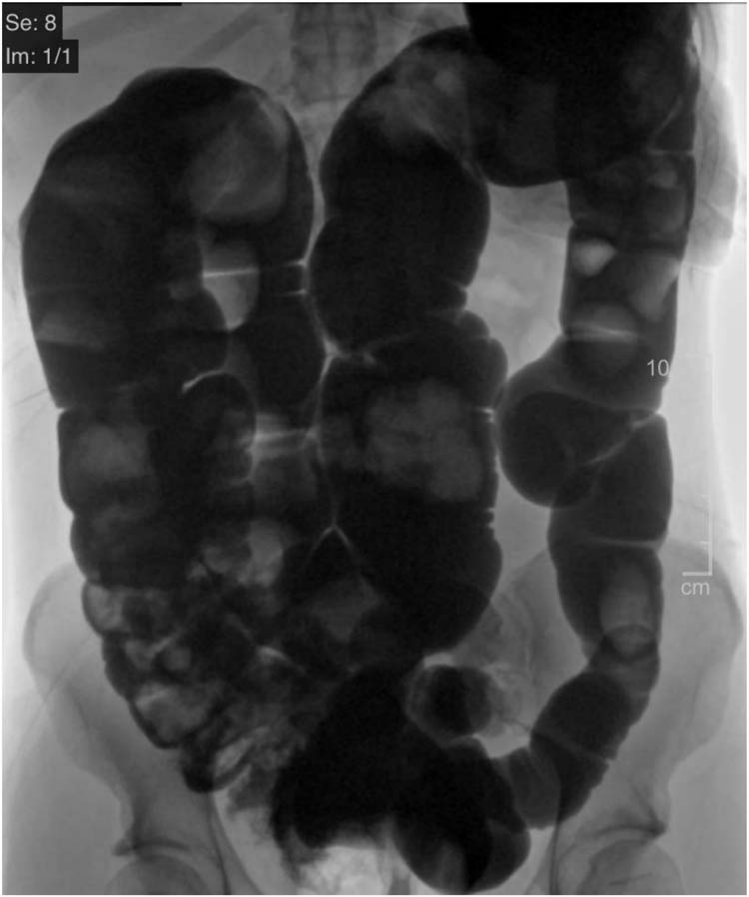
CT Diatrizoate meglumine (DM) enema with air contrast. DM flows in a retrograde manner from the rectum to the cecum without evidence of obstruction or mass.
